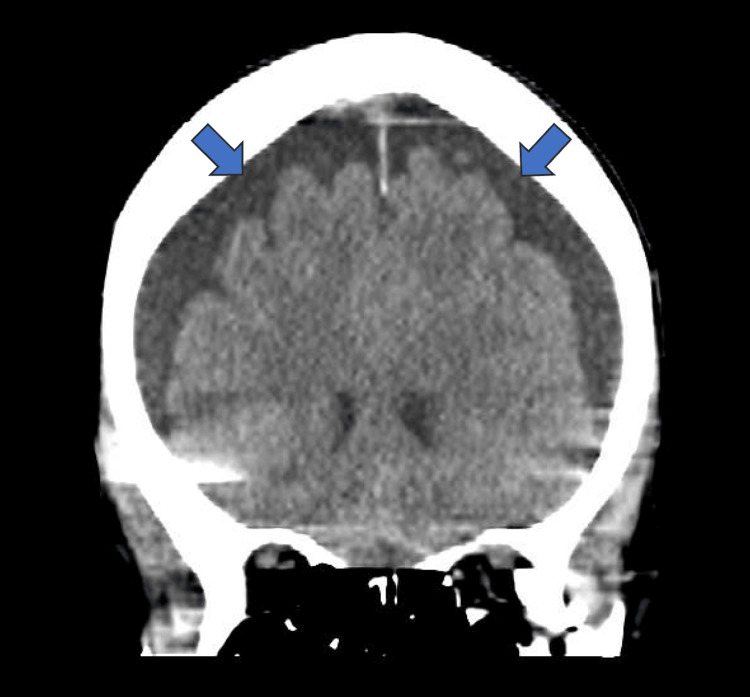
CT scan head showing pneumocephalus in the bilateral anterior convexity (arrows)
